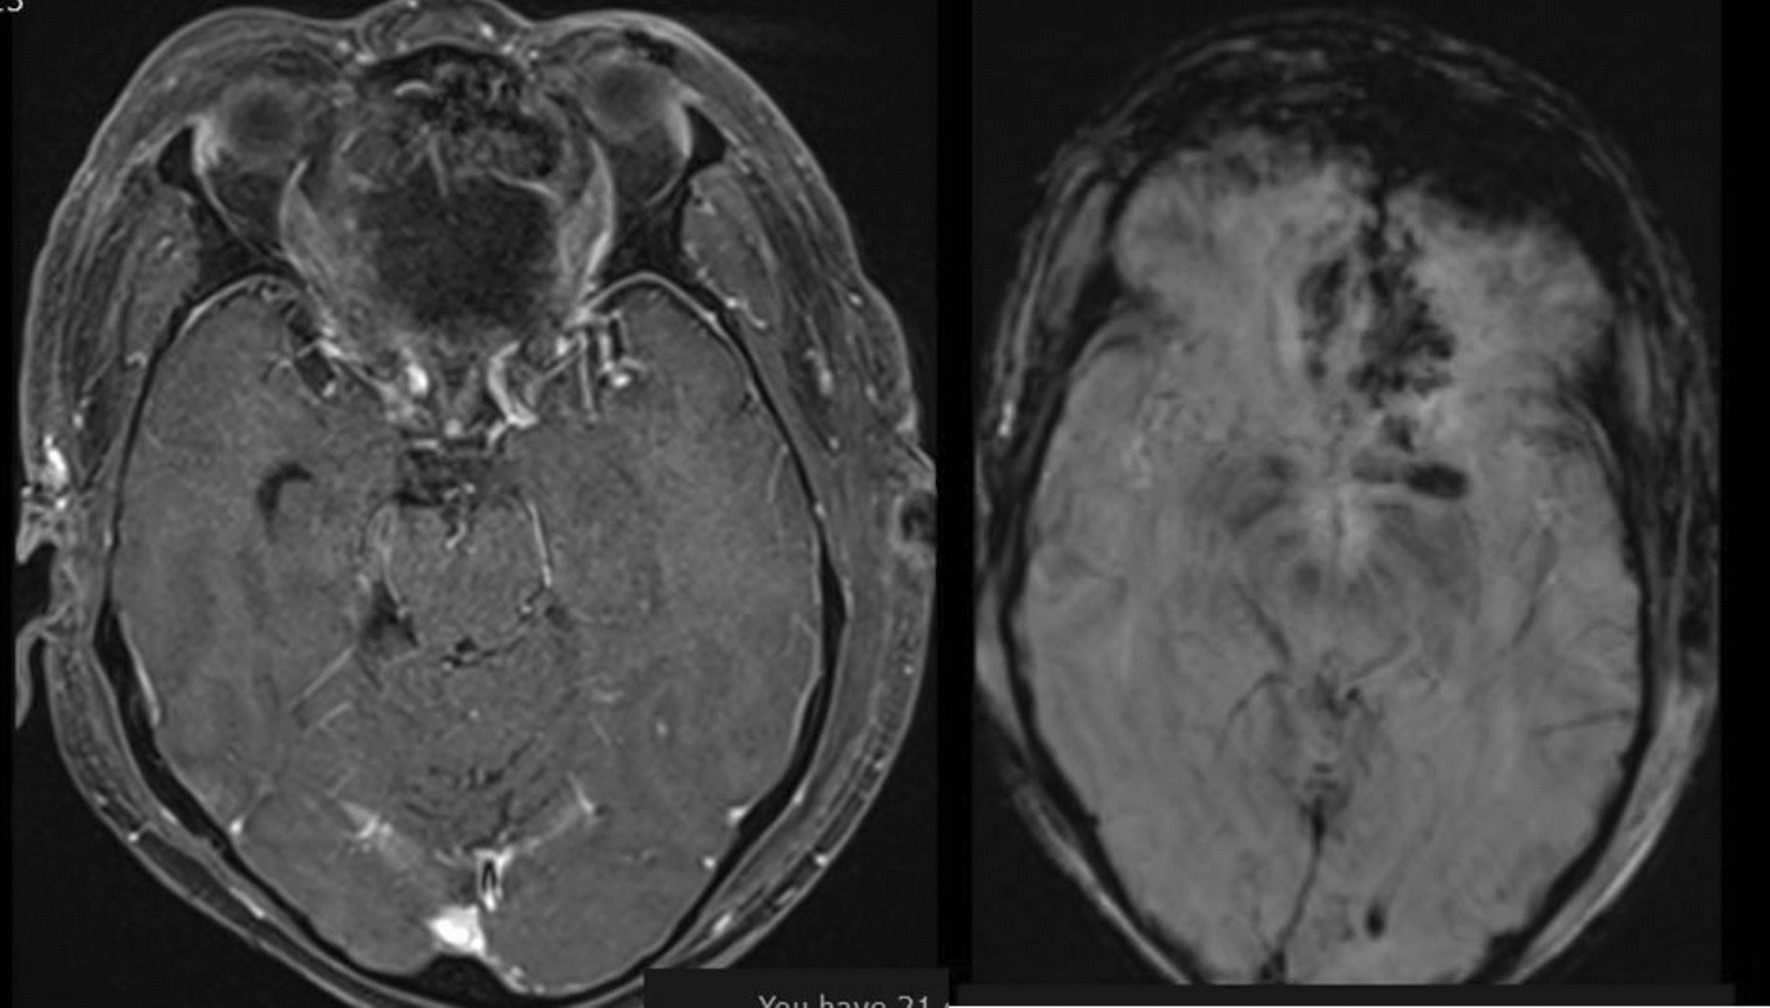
Postoperative post contrast axial magnetic resonance imaging image and susceptibility weighted imaging axial images demonstrating complete resection of the tumor
